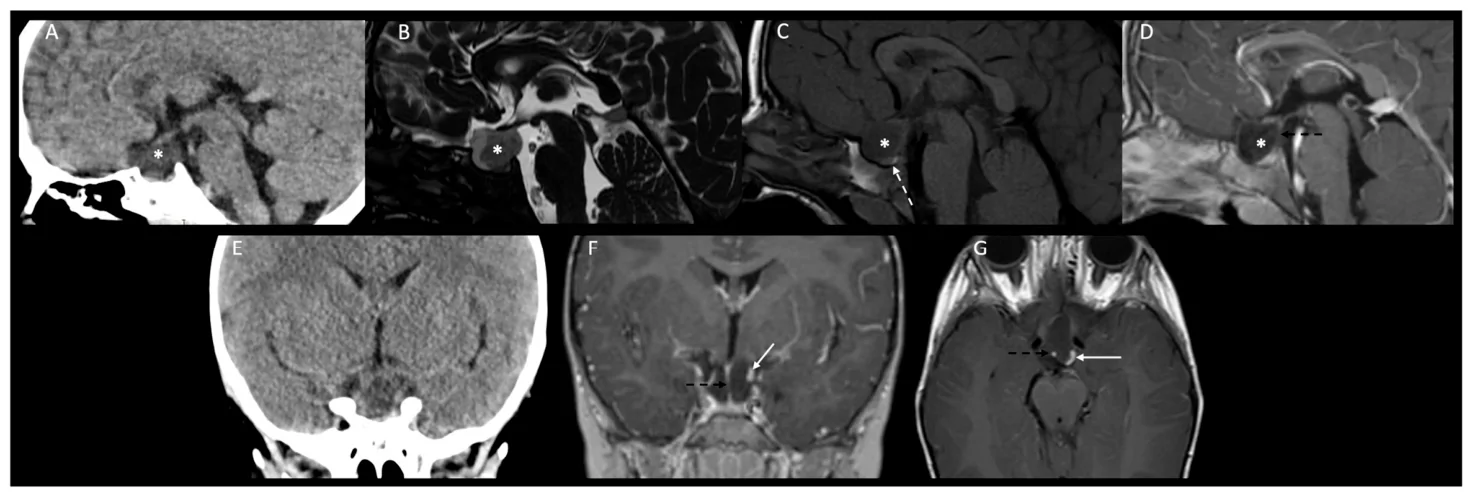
PCP. CT and MR studies of a 7-year-old boy with headache and vomiting. (A) Sagittal CT, (B) sagittal bSSFP, (C) sagittal T1, (D) sagittal contrast-enhanced 3D T1, (E) coronal CT, (F) coronal contrast-enhanced 3D T1, (G) axial contrast-enhanced T1. Sellar/suprasellar expansile lesion (asterisks), characterized by inhomogeneous cystic content and strong enhancement of a solid parietal nodule (arrows). Note the absence of calcifications on CT images (A,E). The pituitary gland is compressed downward (dashed white arrow), and the pituitary stalk appears elongated and stretched posteriorly (dashed black arrows).
